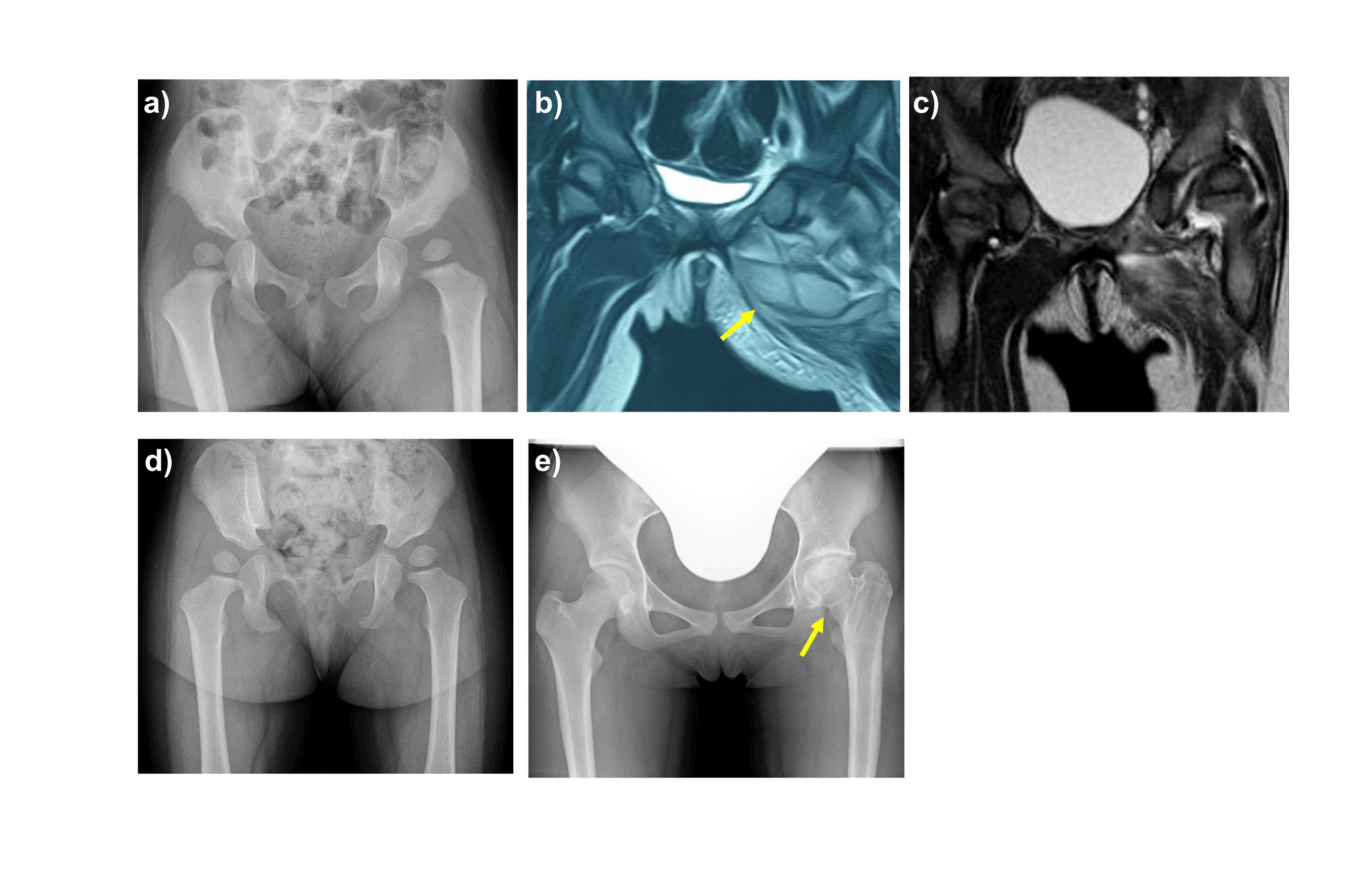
A case of secondary pyogenic arthritis due to pus infiltration into the joint following aspiration a) Lateralization of the left femoral head before treatment.  b) Abscess formation in adductors of the left hip (yellow arrow) in MRI.  c) Joint fluid in the left hip joint after operation of abscess drainage. d) Normalization of the left hip after operation of joint irrigation. e) Femoral head and short neck deformities of the left hip joint (yellow arrow) at the final examination.
